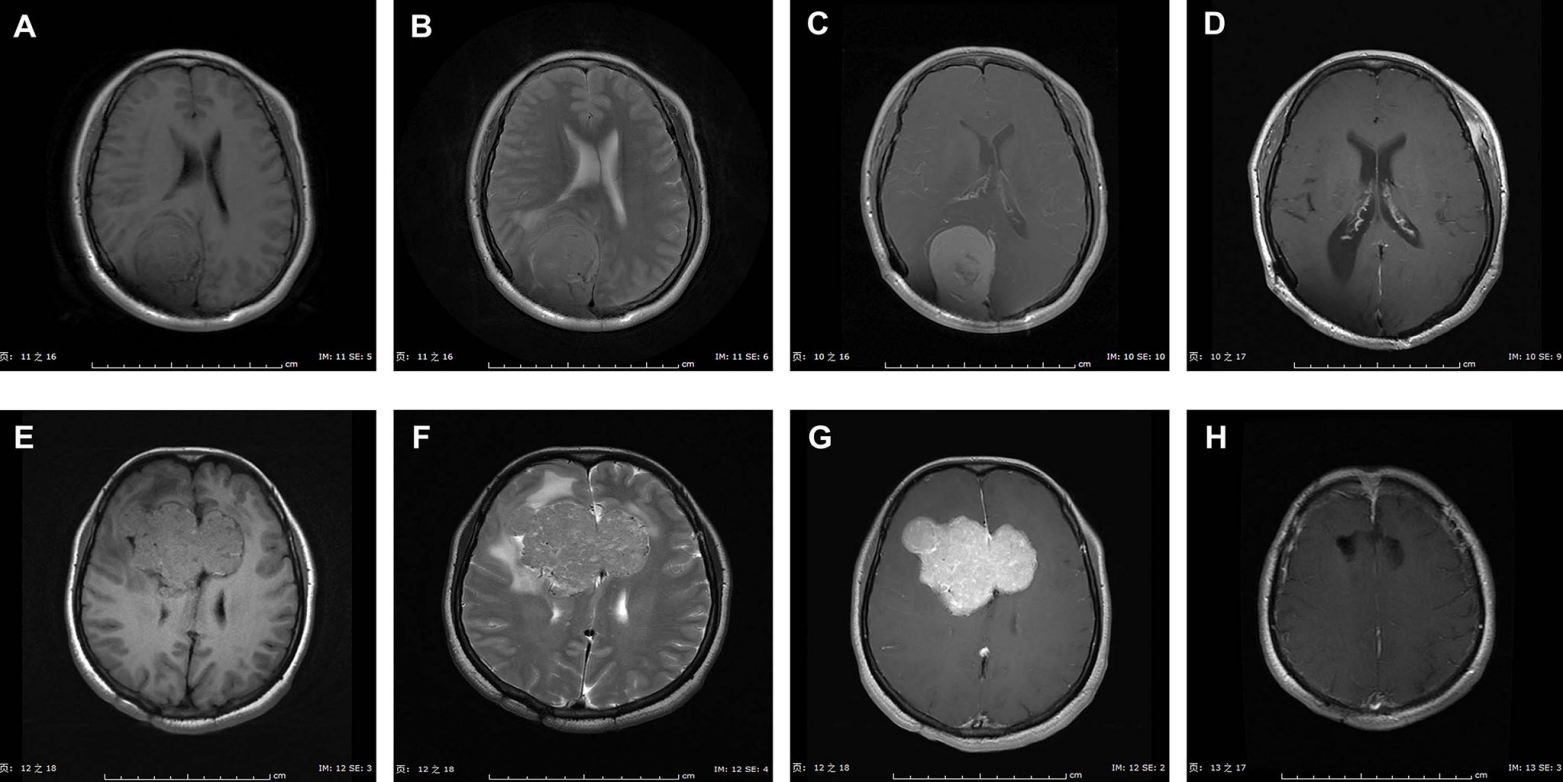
Representative MRI scans of ISFTs in our series. Generally, the signal intensity of the tumor mass was heterogeneous mixed isointense and hypointense on non-contrast T1 and T2 MRI sequences, and marked and heterogeneous enhancement on T1 with gadolinium contrast (T1-Gd) MRI scan. (A–D) MRI scans of a 47-year-old female with ISFT lesion located in right occipital lobe. (A–C) Preoperative axial T1, T2, and T1-Gd MRI scans. (D) Postoperative T1-Gd MRI scan showed a GTR resection. (E–H) MRI scans of a 44-year-old female with ISFT mass originated from callosum and invaded into both sides of frontal lobe. (E–G) Preoperative axial T1, T2, and T1-Gd MRI scans. (H) Postoperative T1-Gd MRI scan demonstrated a GTR resection.
